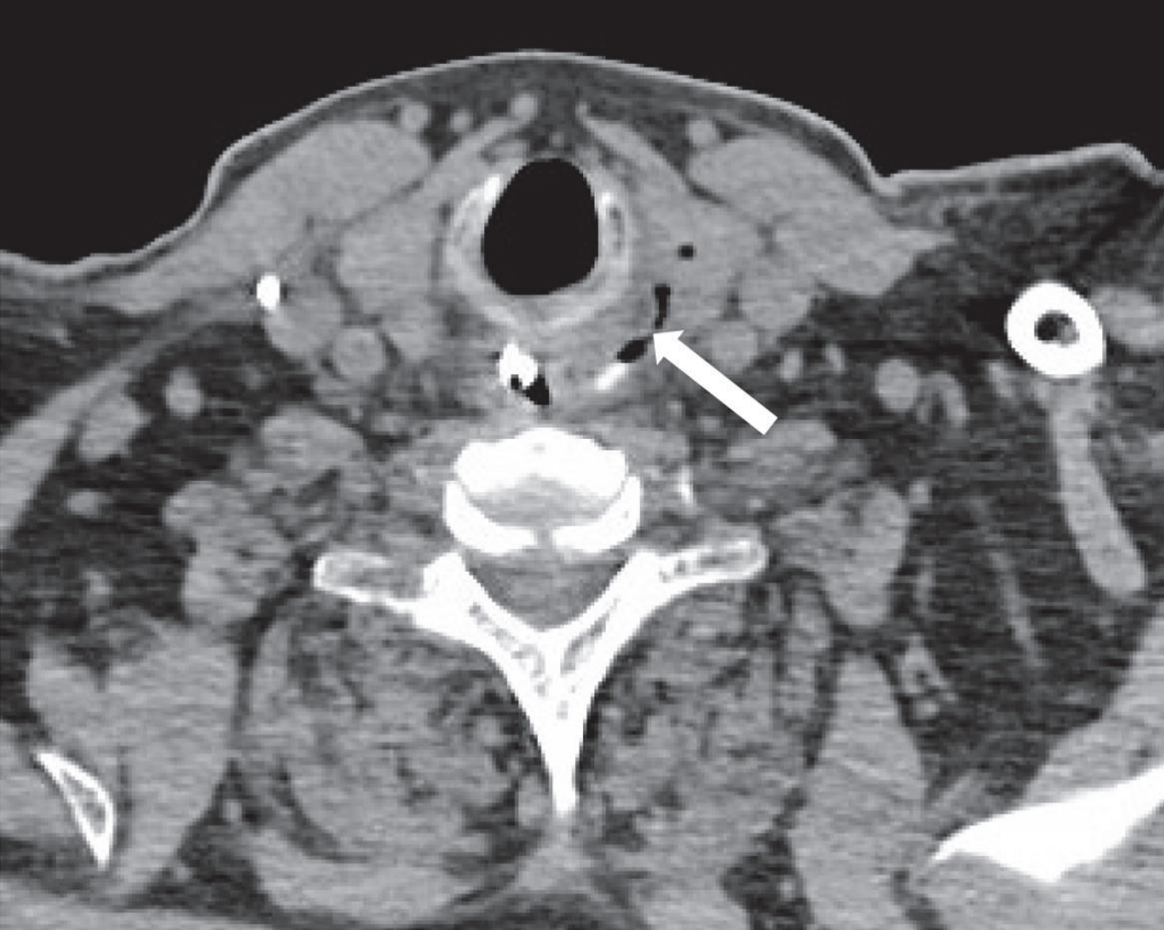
Axial image of neck CT with oral contrast (1 mm slice thickness), showing contrast extravasation at the cricopharyngeal plane within a filiform air tract extending to the posterior border of the left thyroid lobe (arrow).
